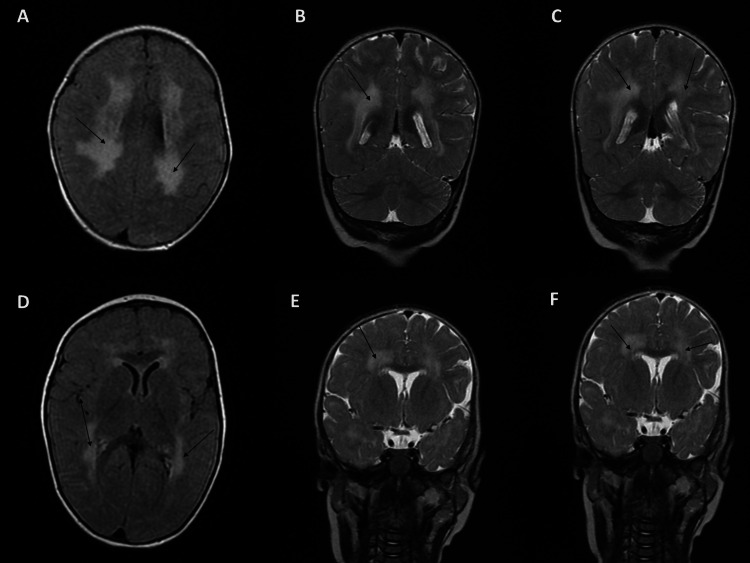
Results of the MRI scan of the case From A to F, the images show changes in the frontoparietal and periventricular white matter of the patient
